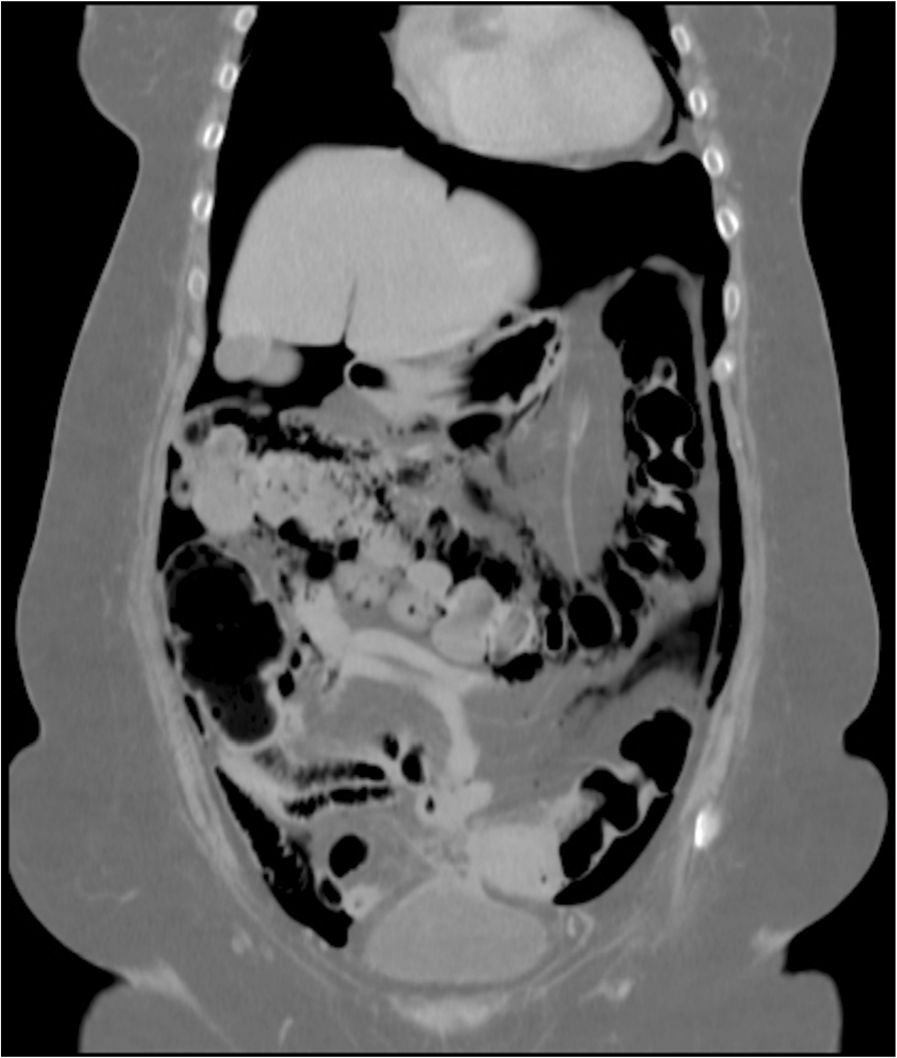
Coronal computed tomography of the distended colon and pneumoperitoneum
